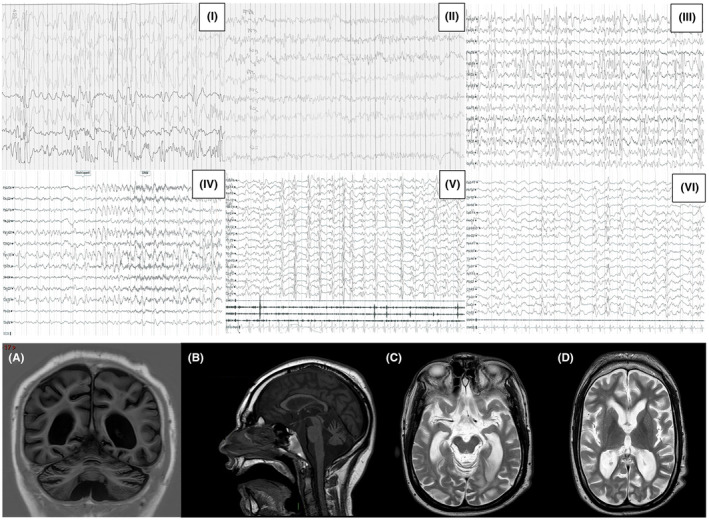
Electroencephalogram (EEG) and magnetic resonance imaging (MRI) evolution of Pt. 2. Panel I‐VI EEG of Pt. 2 from 12 months to 30 years of age (I) sleep EEG registration of Pt.2 (12 months year) shows hypsarrhythmia before the treatment with adrenocorticotropic hormone (ACTH); (II) awake EEG registration of Pt.2 after ACTH therapy shows a bilateral and symmetric 5–6 Hz background activity without epileptic discharges. (III) Awake EEG registration (13 years) showing high voltage, 1.5–2 Hz, spikes‐and‐waves predominantly over the frontal temporal regions. (IV) Awake EEG recording showing a disorganized background with spikes‐and‐waves predominantly on the frontal temporal regions and the start of a generalized seizure with clinical manifestations of staring spell and generalized stiffening. (V‐VI) Awake EEG (24 and 29 years) showing high voltage, bilateral spikes‐and‐spikes, and slow waves on frontal temporal regions only mildly reduced in frequency and amplitude over sequential controls. Pt. 2's MRI at 10 (A and B) and 19 years of age (C and D). (A) (inversion recovery, coronal plane) and B (T1, sagittal plane) show diffuse and generalized brain atrophy and ventriculomegaly with a thin and mildly dysmorphic corpus callosum. Cerebellar and vermian atrophy are also evident. Anterior commissure, optic nerves and olfactory bulbs were intact. (C and D) (T2, transversal plane) show a progression of brain atrophy, mainly in the frontal and temporal lobes (coherent with epileptic discharge localization), with areas of periventricular T2 hyperintensity. An analogous progression of EEG and MRI findings was documented for Pt.1.
